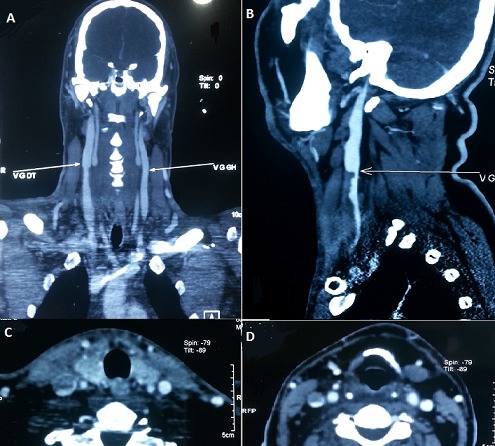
A: CT scan angiography in coronal cut showing the right internal jugular vein thrombosis; B: parasagittal cut showing the thrombosis; C and D: transverse cuts showing the same thrombosis
